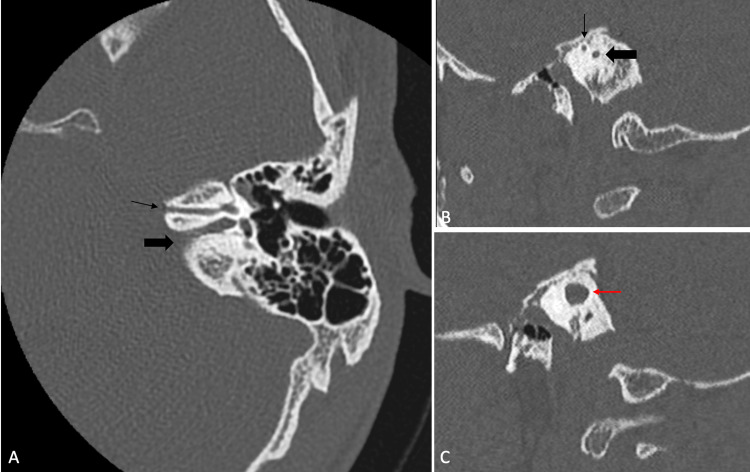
HRCT of the temporal bone in the bone window: (A) axial oblique reformatted image showing a narrow bony canal (thin black arrow) anterosuperior to the stenotic left IAC (thick black arrow), (B) sagittal reformatted images showing narrow left IAC (thick black arrow) with a thin bony canal IAC (thick black arrow) anterosuperior to it, and (3) sagittal reformatted images showing right IAC (red arrow) which is normal in diameter HRCT: high-resolution computed tomography, IAC: Internal auditory canal
